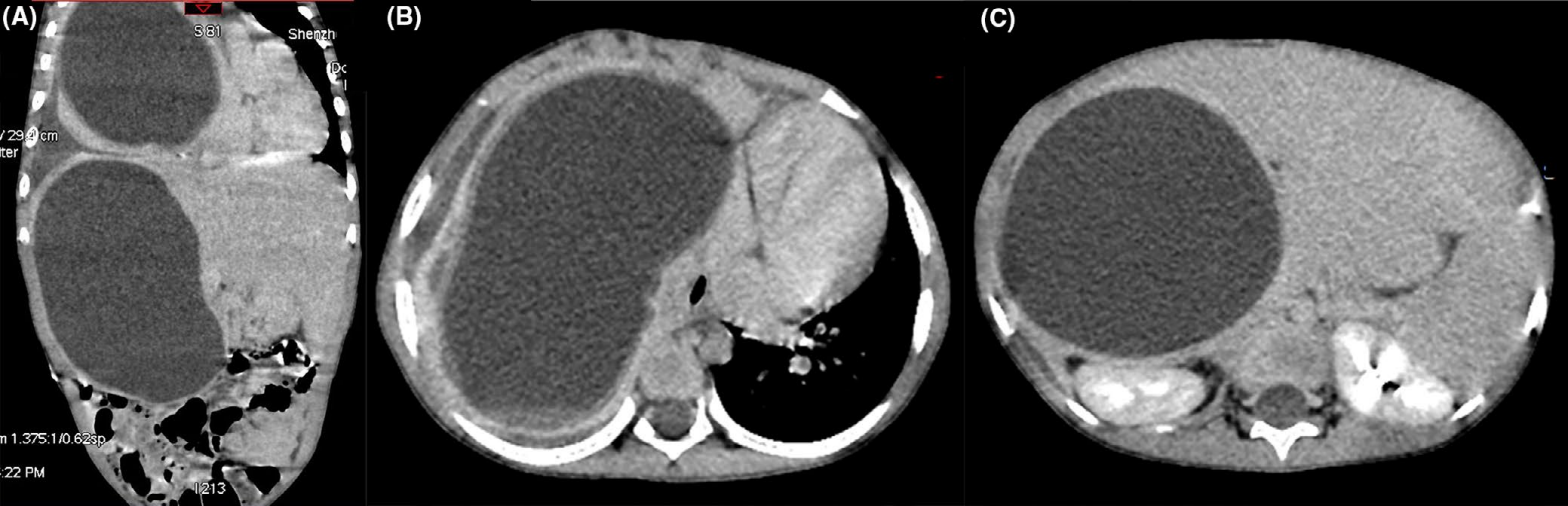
Enhanced computed tomography (CT) scans revealed massive hydatid cysts in the parenchymal portions of the right lung and liver of the patient: A, lung and liver hydatid cysts on the coronal plane, B, lung hydatid cyst on the axial plane, and C, liver hydatid cyst on the axial plane
